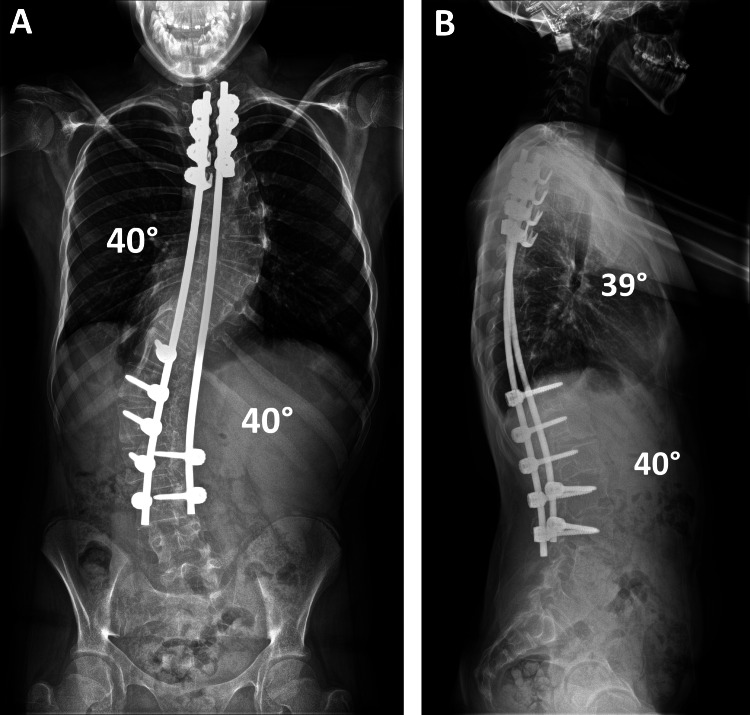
Postoperative radiographs. Posteroanterior radiographs of the spine taken at the last follow-up show a balanced correction of the severe thoracic and lumbar scoliosis, which was maintained over time. Good restoration of the global coronal (A) and sagittal (B) balance of the spine with normal thoracic kyphosis and lumbar lordosis was achieved. At this stage, the patient had returned to her normal level of activities with no complaints of her spine.
